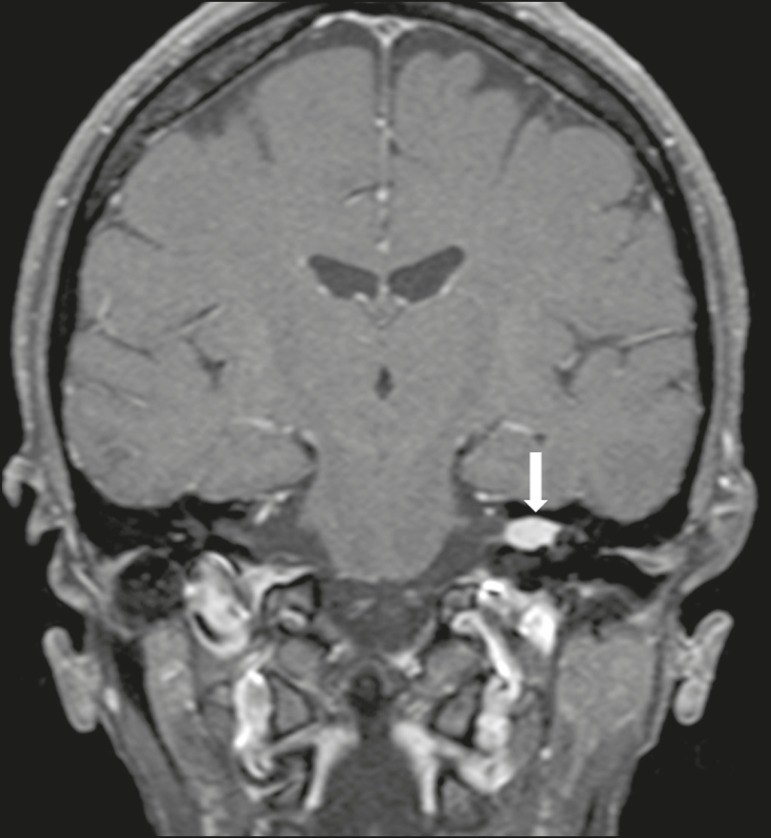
Intracanalicular schwannoma. Contrast-enhanced coronal T1-weighted
